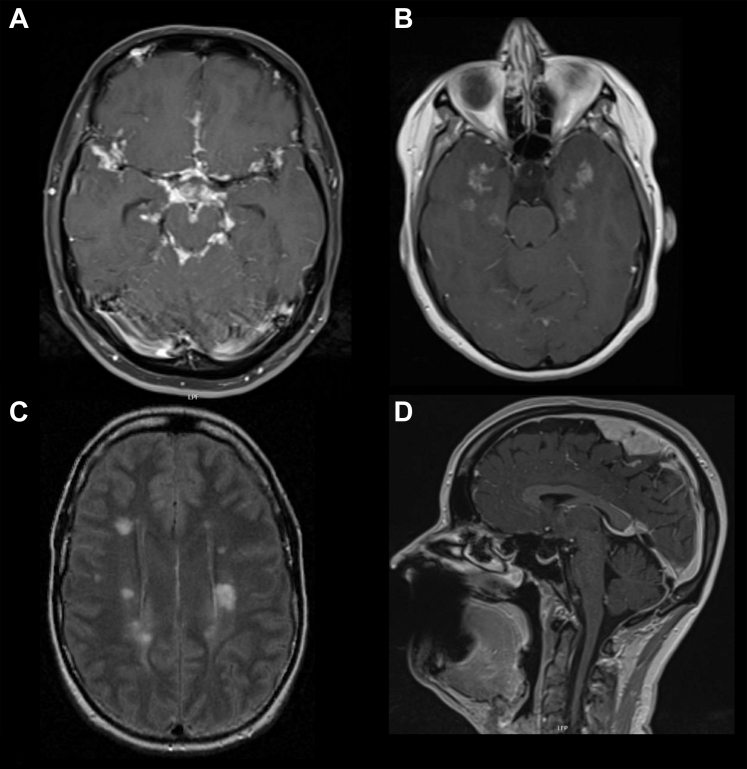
Parenchymal and meningeal involvement in neurosarcoidosis. A: 32 yo female with fatigue and headache, biopsy confirmed sarcoidosis MRI T1 + Gd showing leptomeningeal enhancement. B: 28 yo female with biopsy confirmed sarcoidosis and migraine, cranial MRI, T1 + Gd showing parenchymal enhancement in temporal lobe. C: 40 yo female with initial diagnosis of multiple sclerosis, MRI FLAIR showing white matter lesions, later diagnosed as neurosarcoidosis based on biopsy confirmed sarcoidosis. D: 37 yo female with focal epilepsy and extra-axial lesion suspected to be meningeoma or metastasis, MRI T1 + Gd showing homogeneous enhancing mass adjacent to the falx, which proved to be definite neurosarcoidosis after resection.
